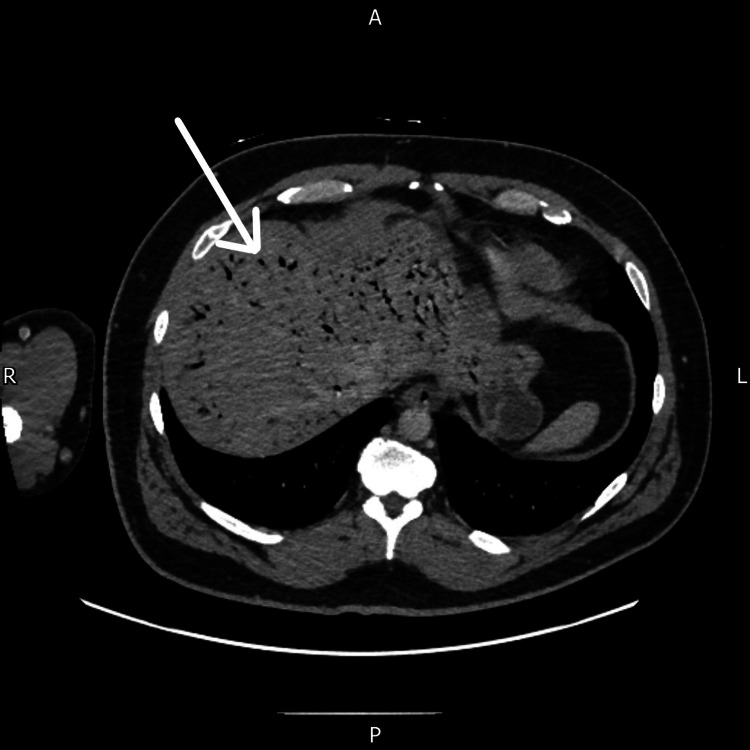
CT scan of the abdomen and pelvis showing extensive gas accumulation within the hepatic-portal vein branches, as observed in the axial view
